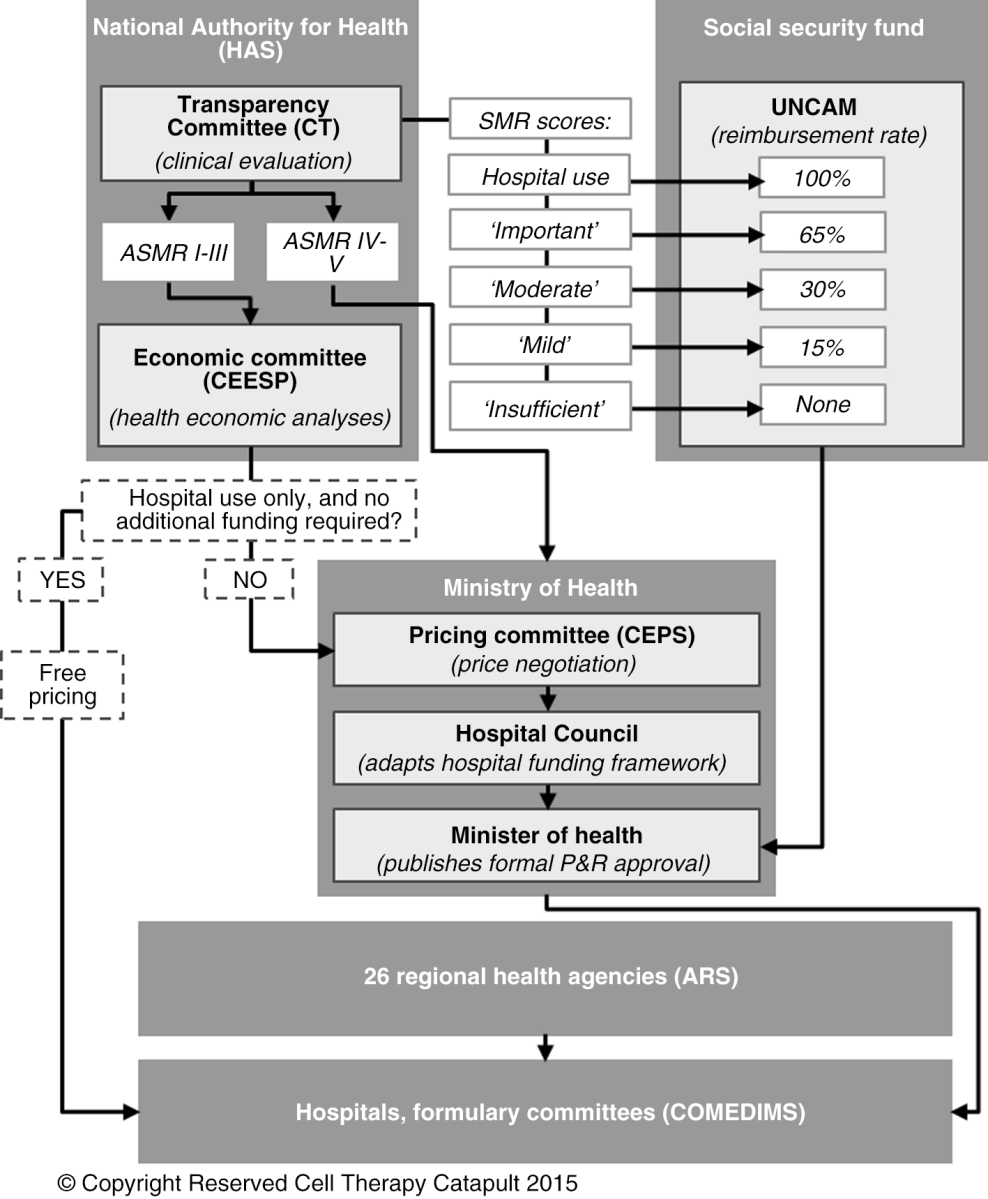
Market access process for drugs in France. ARS: Regional Health Agencies; COMEDIMS: Committee for Medicines and Sterile Medical Devices; CEESP: Economic and Public Health Assessment Committee; CEPS: Economic Committee for Health Care Products; CT: Transparency Committee; HAS: French National Authority for Health; P&R: pricing and reimbursement; SMR: actual benefit; ASMR: improvement in actual benefit; UNCAM: National Union of Health Insurers.
